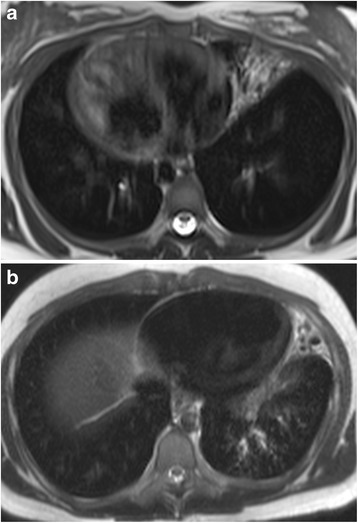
Transversal MR images of a 14–year-old girl with PCD and *situs viscerum inversus* showing an area of consolidation in the middle lobe (a), and of a 15–year-old boy with CF showing an area of consolidation in the lingula and sparse bronchiectasis in the left lower lobe (b)
